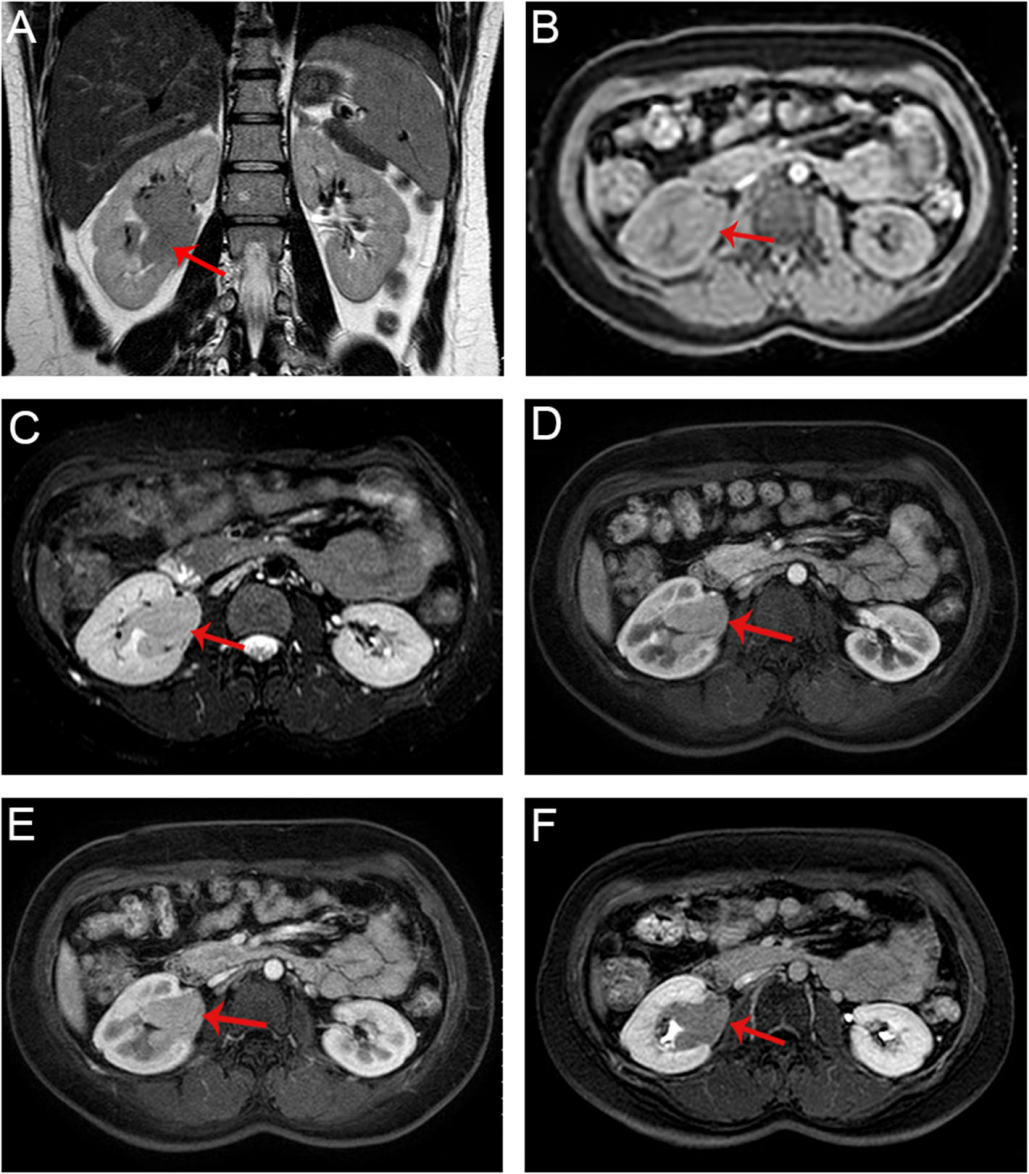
Contrast-enhanced abdominal MRI of the lesion (red arrow) in case 1. (A) Coronal T2-weighted image; (B) Transverse T1-weighted with fat suppression (C) Transverse T2-weighted with fat-suppression; (D) Transverse T1-weighted with fat-suppression in arterial phase; (E) Transverse T1-weighted with fat-suppression in portal phase; (F) Transverse T1-weighted with fat-suppression in delayed phase
